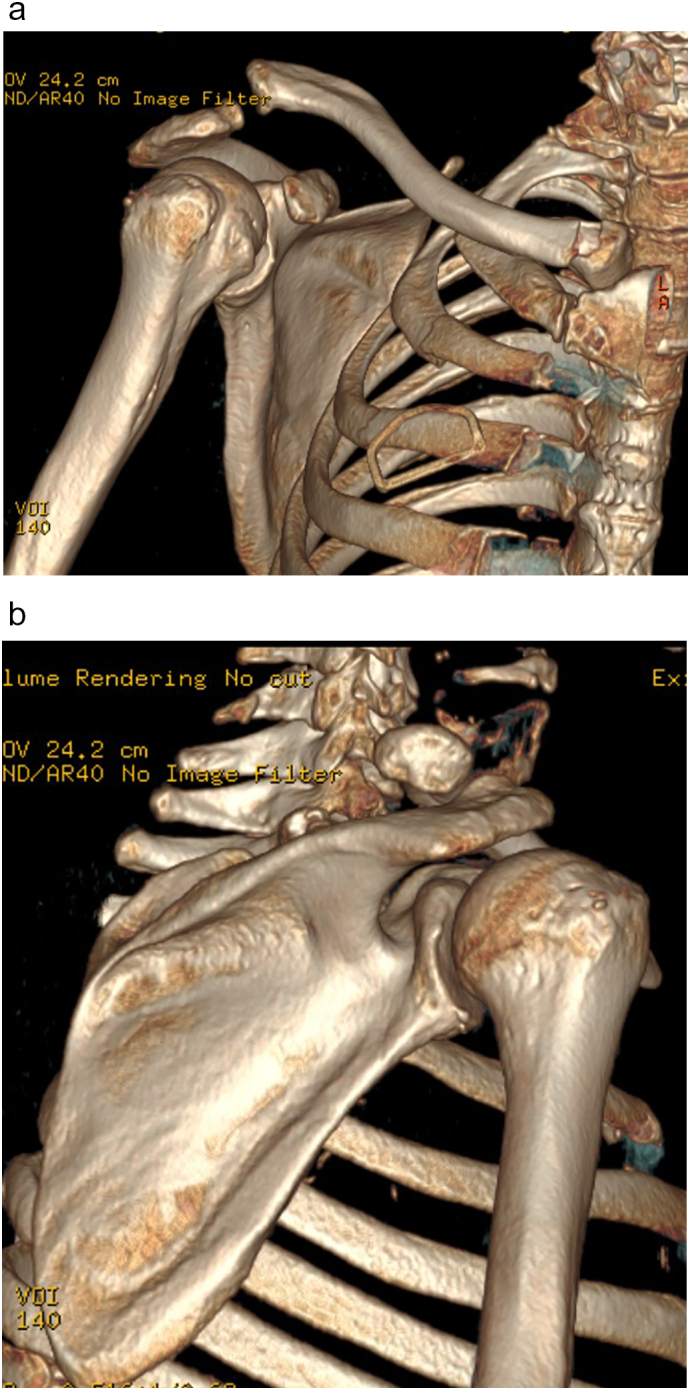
Computed tomography scan 3D reconstruction confirming a proximal clavicle conminuted fracture and ipsilateral 2nd and 3rd rib fractures (A) and posterosuperior displacement of clavicle at the acromioclavicular joint (B).
